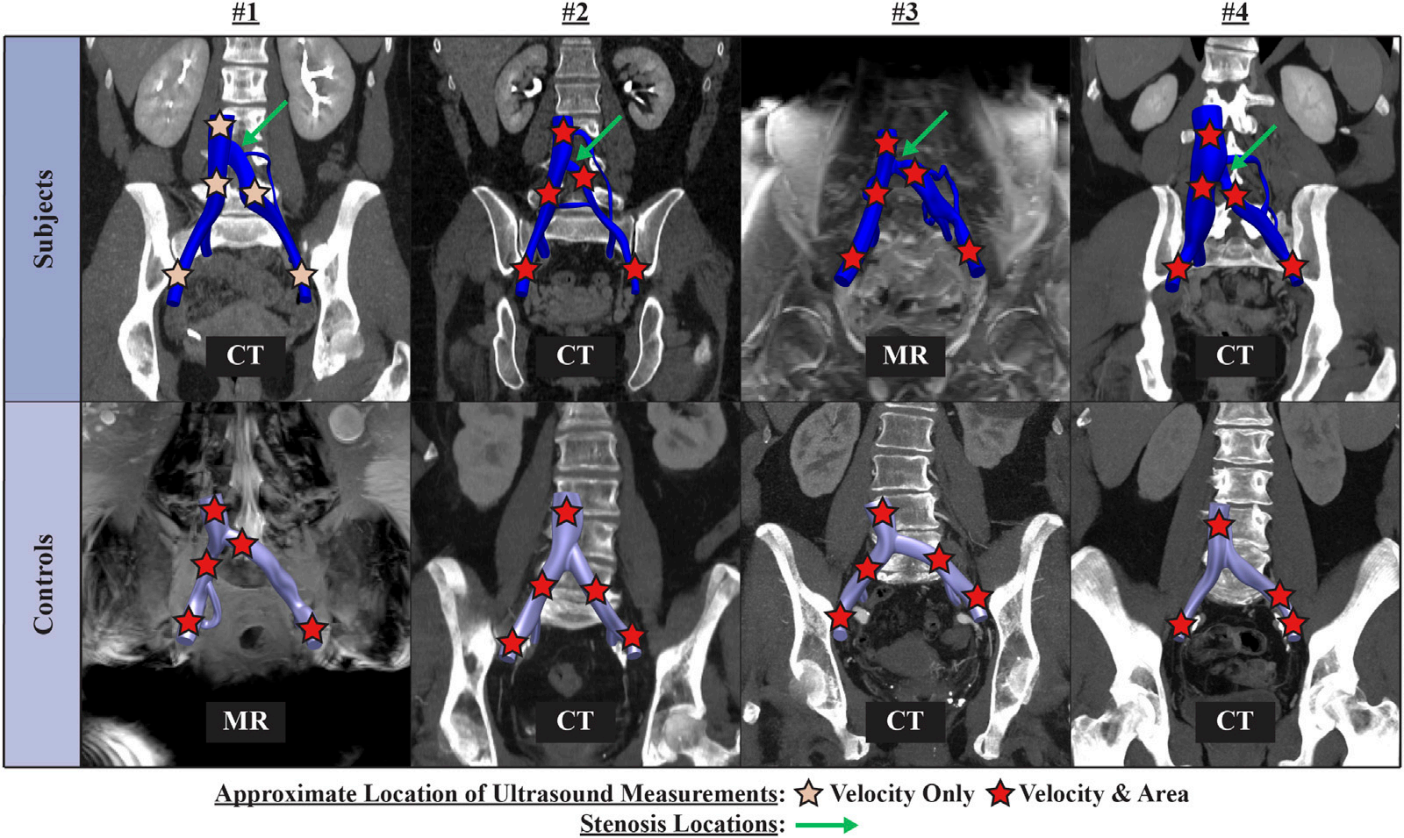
Overview of contrast-enhanced CT or MR image data and approximate location of ultrasound measurements for each patient.
